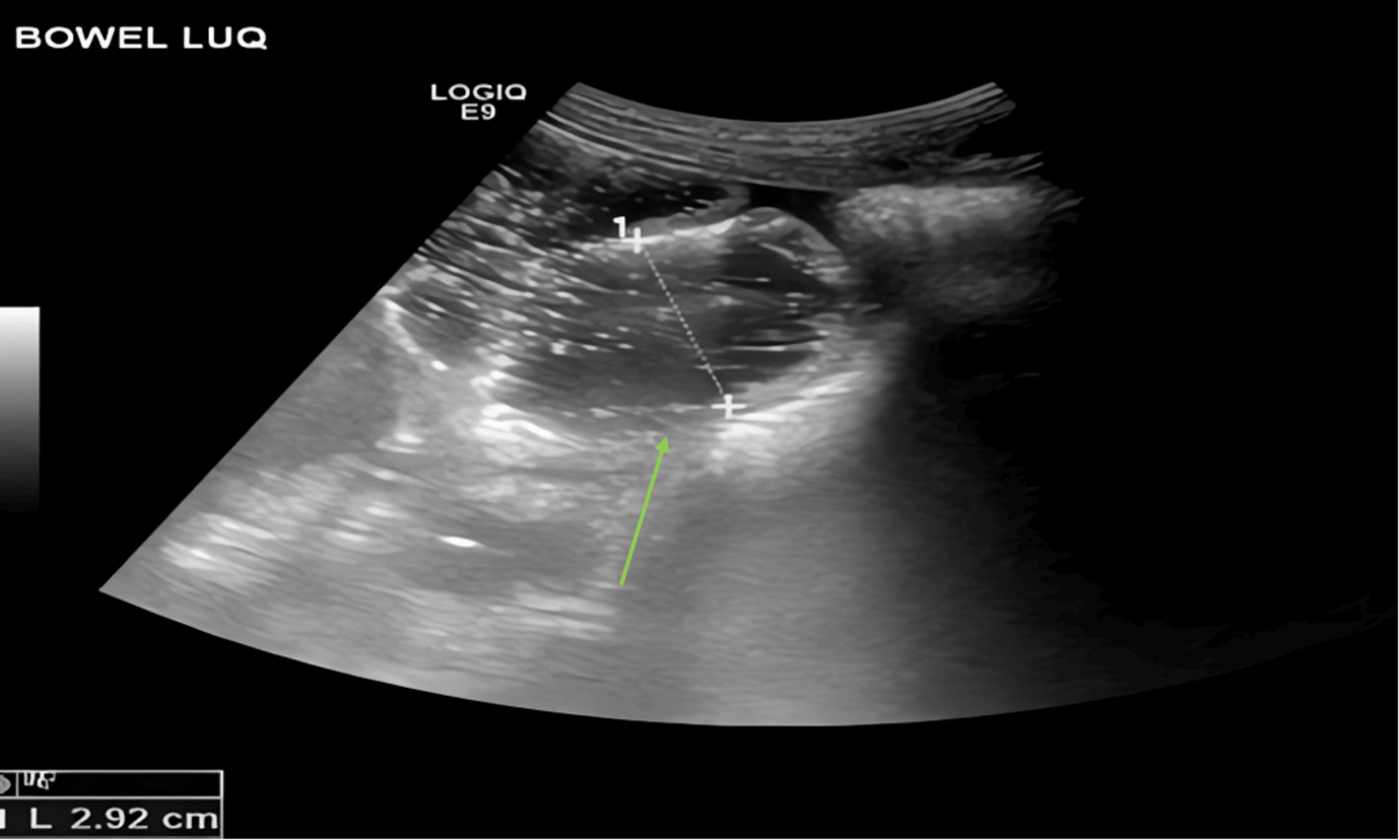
Ultrasound of the Left Upper Quadrant Ultrasound demonstrating markedly distended bowel loops (green arrow) and possible pneumatosis in the left lower quadrant (initial imaging).
